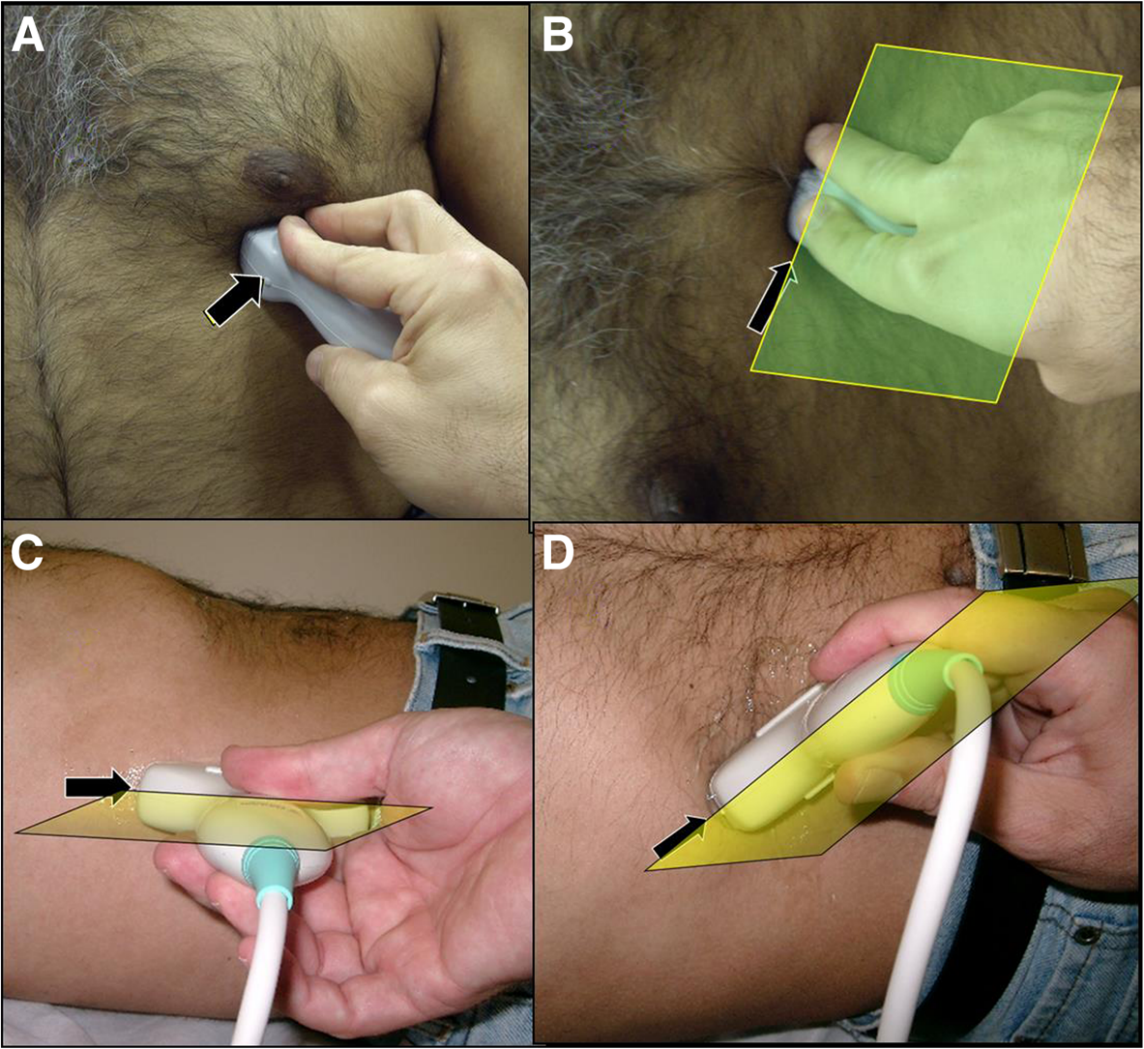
Acute care physicians use a global clinical ultrasound examination approach and may examine different anatomical regions in the same patient. The pointer of the ultrasound probe should be routinely directed proximally or to the right side of the patient even in echocardiography or oblique views. The figure demonstrates four different views, the cardiac apical four chamber view (a), the cardiac subcostal view (b), the coronal abdominal and thoracic view (c), and the oblique abdominal view (d). The marker is pointing proximally or to the right side of the patient
